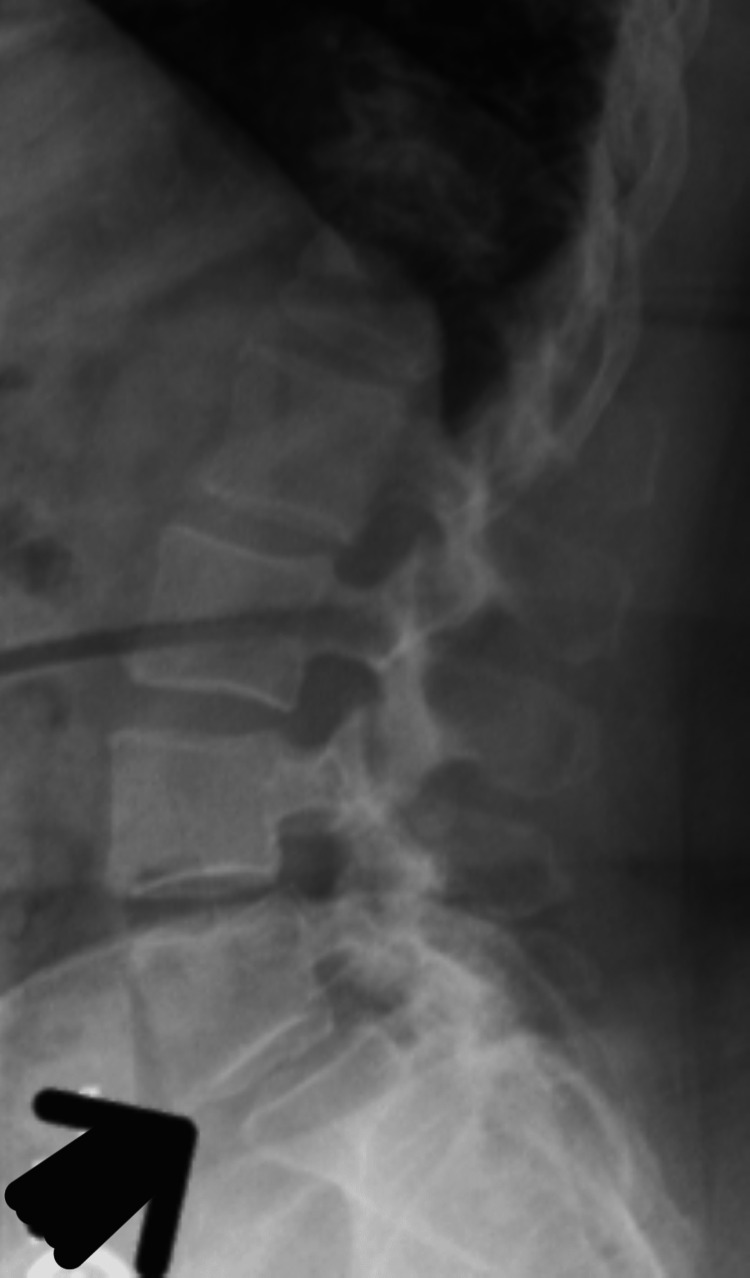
The x-ray image of the lumbosacral region. The image shows multilevel degenerative changes in the fourth lumbar to the first sacral vertebrae and a grade 1 anterolisthesis between the fifth lumbar vertebrae and the first sacral vertebrae (big arrow).
